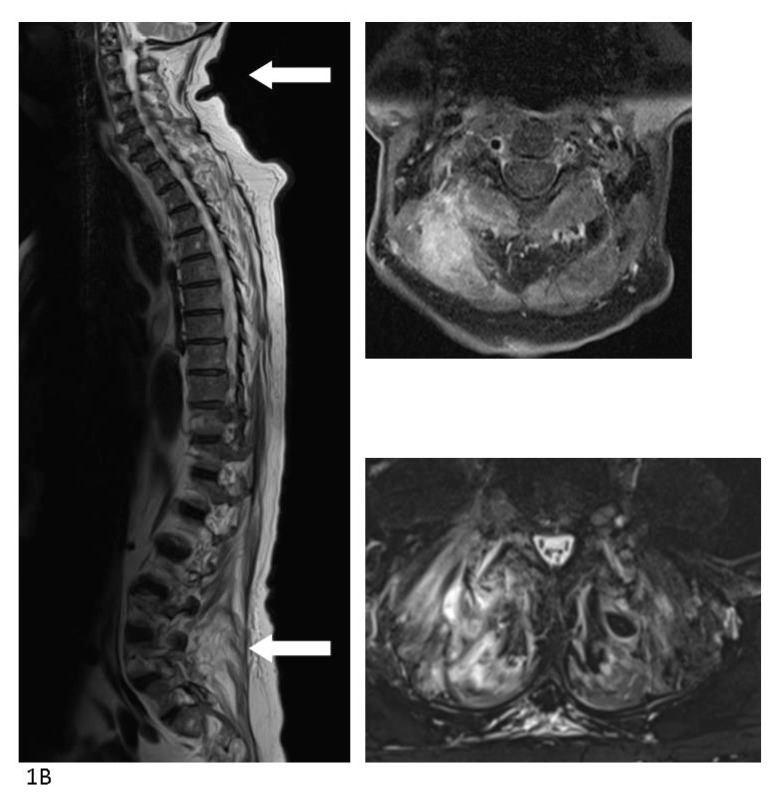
A 64-year old man was admitted to a local hospital with sudden onset of fever, difficulty breathing, and chronic chest and back pain. The patient’s prior medical history included stroke with minor sequelae, well-regulated hypertension, moderate alcohol abuse, and sleep apnea. He was on dual anti-hypertensive medication, muscle relaxants, and blood-thinner. The patient lived alone receiving help for cleaning and shopping. The patient was admitted septic and not fully oriented with a temperature of 39.6 °C, blood pressure of 74/50 mmHg, 95% saturation on 2L O_2_. Following regional guidelines, treatment with piperacillin/tazobactam was initiated. A computerized tomography (CT) with intravenous (IV) contrast performed on the suspicion of pyelonephritis/abdominal focus revealed no pathological findings. Endocarditis was ruled out by trans-esophageal echocardiography. His blood cultures grew methicillin susceptible *Staphylococcus aureus* (MSSA) and dicloxacillin and later rifampicin were added to the treatment. No longer septic, the patient was moved to the section for infectious diseases at the regional university hospital. The patient’s main complaint was diffuse pain in the entire back, resulting in the working diagnosis of bacterial (MSSA) spondylitis. A fluorine-18 fluoro-2-deoxy-d-glucose (^18^F-FDG) positron-emission tomography (PET) combined with a diagnostic CT scan with iv contrast was performed. High FDG uptake was found in the left shoulder and several muscular loci in the musculi semispinalis capitis, piriformis, and gluteus maximus dexteres as well as the lumbar part of the erector spinae, but no signs of spondylitis. FDG uptake in the transverse colon prompted a high suspicion of malignancy. (A) The PET/CT scan was supplemented a few days later by a magnetic resonance imaging (MRI) with iv contrast which showed significant inflammation of the muscles—most intensely in the neck and lumbar region and again no signs of spondylitis. (B) A slow recovery on physical therapy and iv antibiotics was interrupted by sudden deterioration possibly due to aspiration pneumonia with admission to the intensive care unit for several days. Colonoscopy with biopsy ruled out colonic cancer but found a high-grade neoplasia of the colon and the surgeons made plans for further work-up. Slow but steady recovery continued and a second MRI with iv contrast four weeks after the initial showed less inflammation of all muscular foci. At that point, during rounds, the patient revealed that he regularly received acupuncture of the entire back and shoulder due to muscular tensions and that he had done so for several years. The last session of acupuncture was performed six days prior to the initial admission at the local hospital. He described that the acupuncture was performed at the local physiotherapist, and that he during the last session had placed 24 needles from the neck down to the buttocks. No anti-septics were used during the session. The patient was discharged after eight weeks of iv antibiotic treatment with another eight weeks of oral antibiotics for ambulant follow-up with the department for infectious diseases and municipal physiotherapy. Both local and systemic infections following acupuncture have been reported previously with different organ manifestations and the causative microorganism is most commonly *Staphylococcus aureus* [1,2,3]. With the widespread use of acupuncture for very different medical conditions in settings with no supervision or hygiene regulation, the use of this procedure should be investigated in cases with systemic infection or in cases with several infectious loci, especially when the imaging does not match the initial diagnosis.
